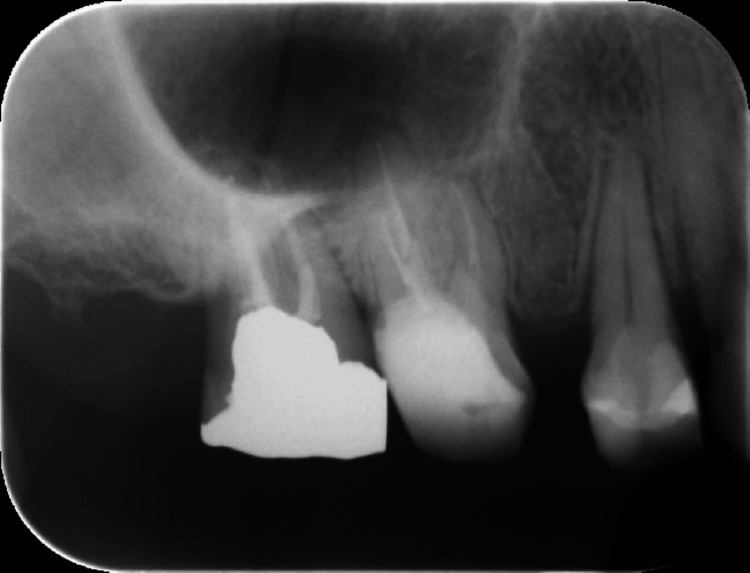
Periapical radiograph Periapical radiographs revealed a slight radiolucent area at the palatal root apex of the upper right first molar.
